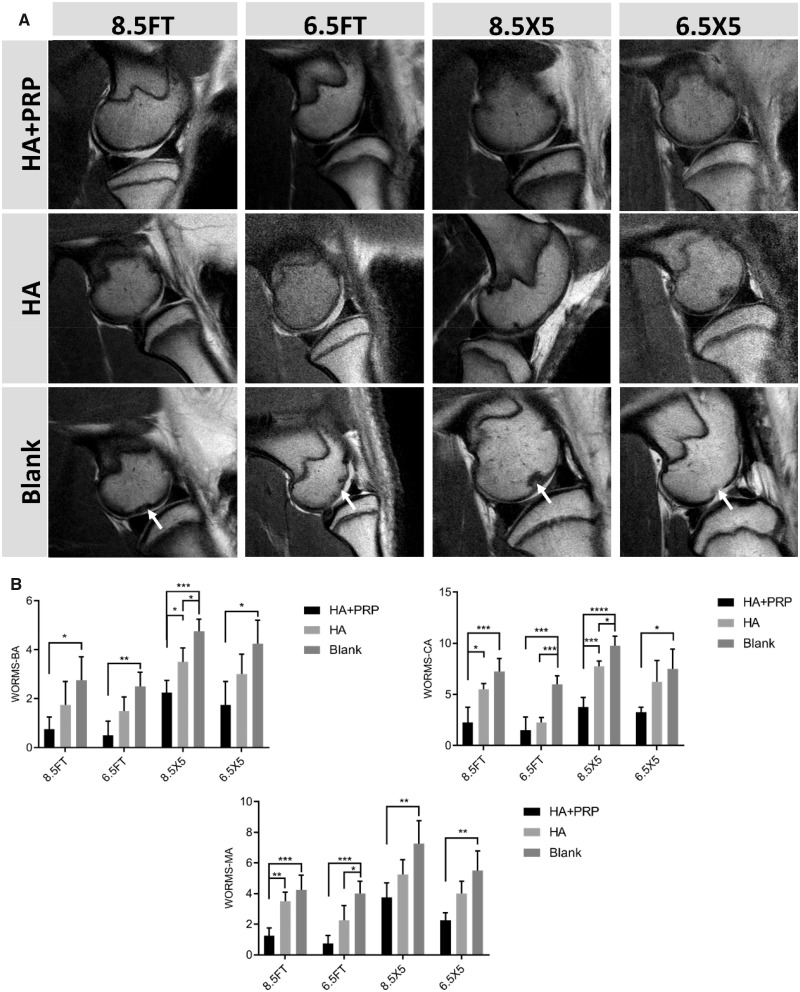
(A) The high-resolution magnetic resonance imaging of articular cartilage in the sagittal plane. From left to right, the arrows indicated abnormal subchondral bone marrow signal, diffused cartilage erosion, subchondral bone defect and cartilage defect. (B) The MRI ICRS-WORMS scoring of repaired tissue. WORMS-BA, WORMS-Bone attrition; WORMS-CA, WORMS-Cartilage; WORMS-MA, WORMS-Marrow abnormality. The results were represented as mean ± standard deviation. P *<* *0.05; *P *<* *0.01; P *<* *0.0005; *P *<* *0.0001
